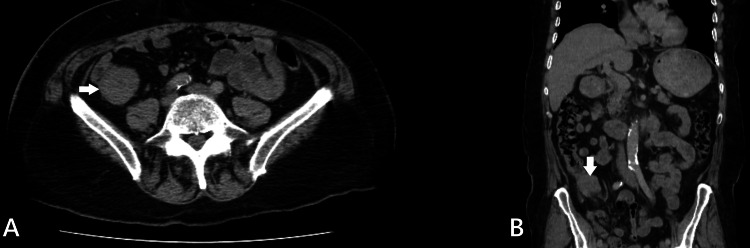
Abdominal computed tomography findings (A) The axial plane of the abdominal CT scan shows a focal thickening of the ileocecal valve associated with fat stranding and no perilesional lymph nodes (white arrow); (B) The coronal plane of the abdominal CT scan shows the same finding (white arrow).
